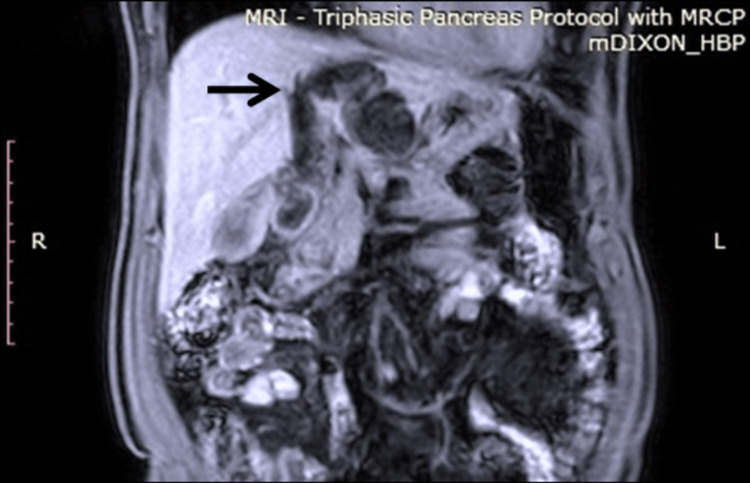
T1 Dixon coronal section of MRI abdomen. The cystic lesion is noted to have communication (arrow) to the dilated left hepatic duct.
